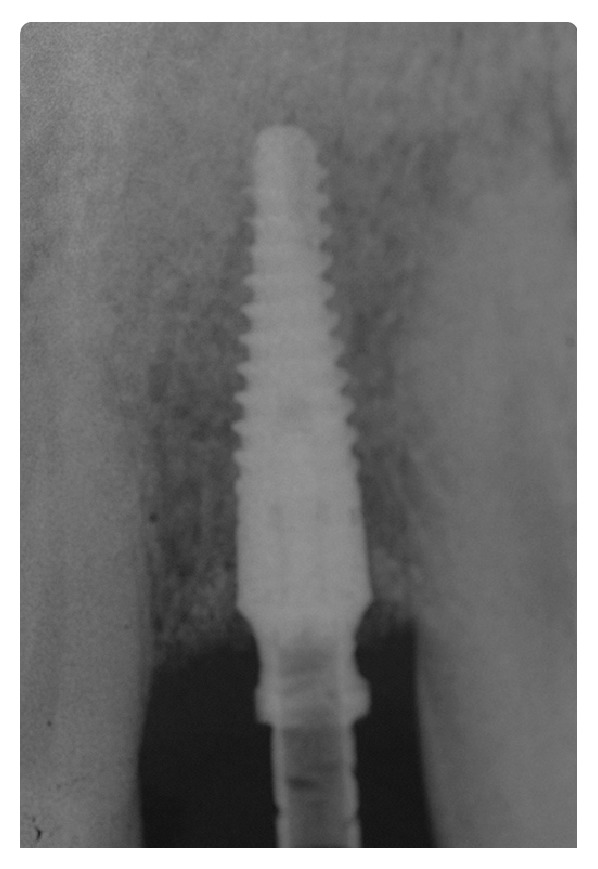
Periapical Rx immediately after implant placement with provisional screw anchorage.
